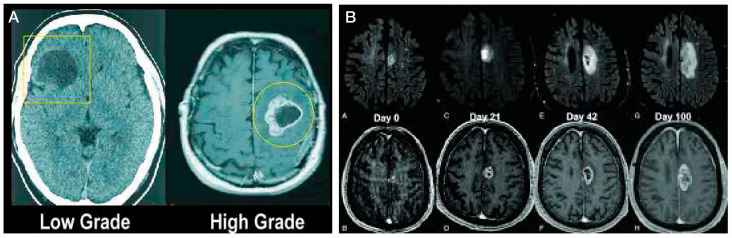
(A) Low grade cerebral gliomas typically show only a low-density area (dark area) in MRI scans, whereas high grade gliomas usually show more contrast enhancement (white on the outside and necrosis in the middle (looks black on the MRI). (B) Rapid glioblastoma cancer progression in size over a 100-day period.
